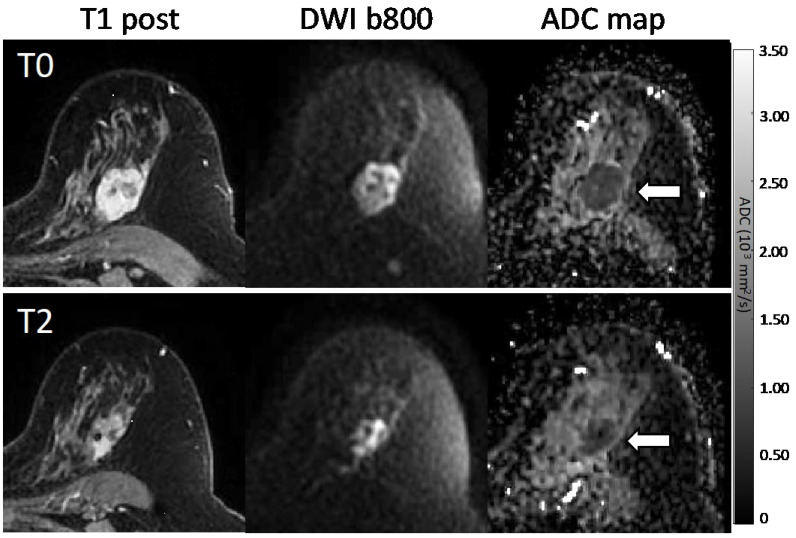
Serial MR images in a 57-year-old patient with a 3.2 cm HR+/HER2− high-grade invasive ductal carcinoma with non-pCR outcome. Shown are representative matched slices from the dynamic contrast-enhanced (DCE) early post-contrast scan, diffusion-weighted image (DWI; *b* = 800 s/mm^2^), and quantitative apparent diffusion coefficient (ADC) map for MRI examinations at the pre-treatment (T0, top) and mid-treatment (T2, bottom) timepoints. Between T0 and T2, the subject showed a decrease in tumor volume on DCE from 12.7 cc to 5.2 cc, while the mean tumor ADC remained unchanged at 0.92 × 10^−3^ mm^2^/s.
